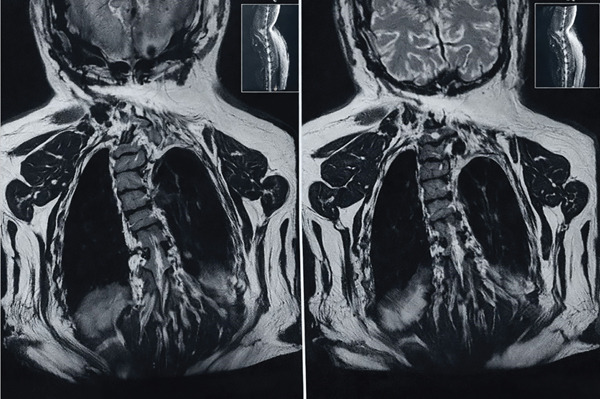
T2‐weighted axial MRI of the cervical spine showing evidence of diastematomyelia at the C3–C4 level.
